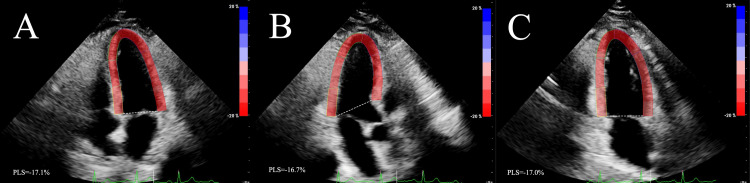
The measurement of PLS in the apical four-chamber (A), apical long-axis (B), and apical two-chamber (C) views from an RA patient. Abbreviations: PLS, peak longitudinal strain; RA, rheumatoid arthritis
